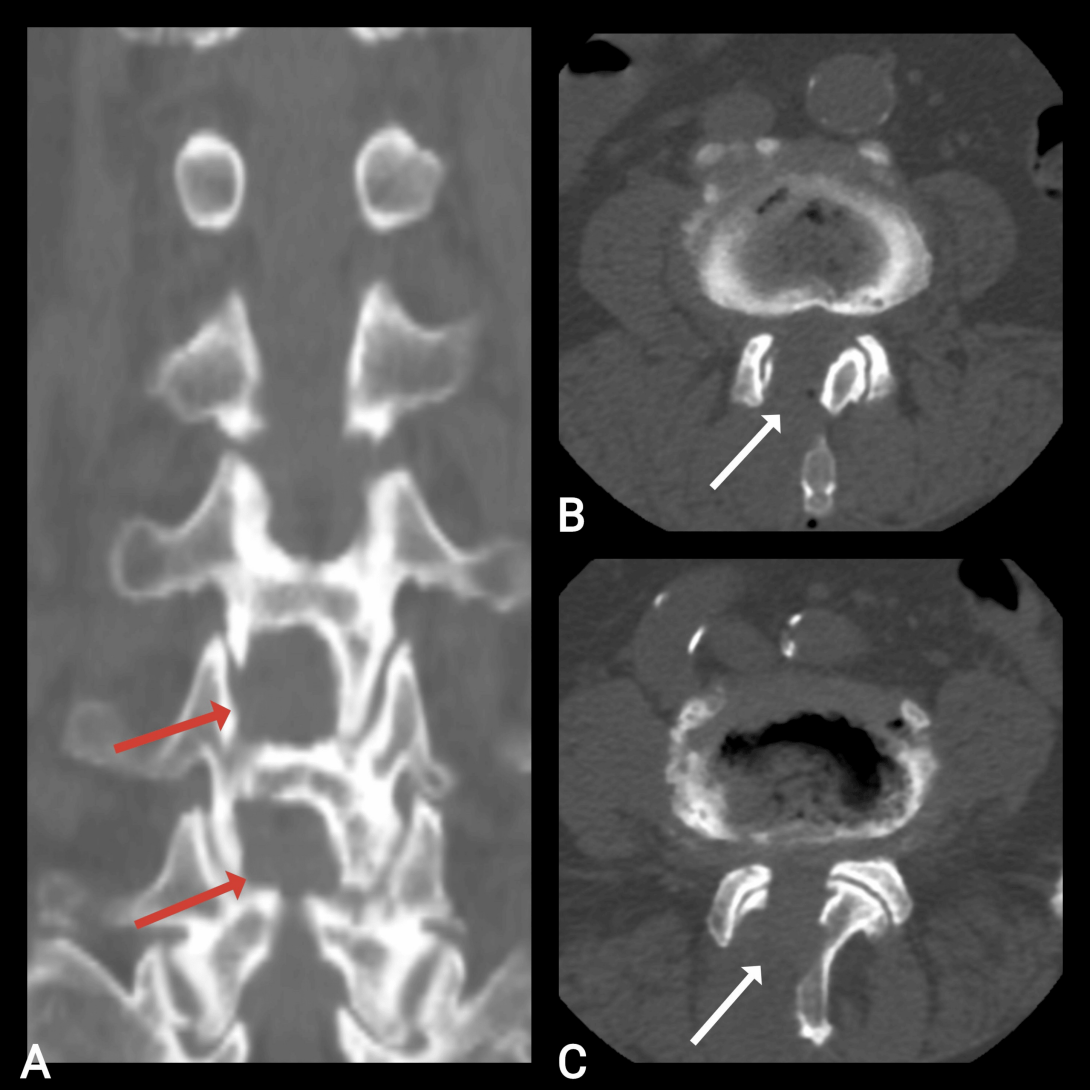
Postoperative CT scan of an 81-year-old male showing the performed decompression in the coronal plane (red arrow) and axial plane (white arrow) at the L3-L4 and L4-L5 levels. A: Coronal plane; B: axial plane at the L3-L4 level; C: axial plane at the L4-L5 level.
